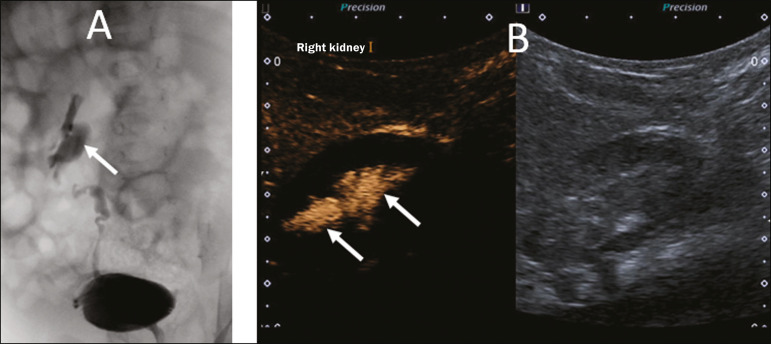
A three-month-old patient undergoing investigation of vesicoureteral reflux. A: Voiding cystourethrography showing vesicoureteral reflux with filling of the renal pelvis and calyces by contrast medium (arrow). B: Voiding urosonography in the contrast-specific mode, showing reflux of microbubble contrast medium (injected through the bladder catheter) to the renal pelvis (arrows).
